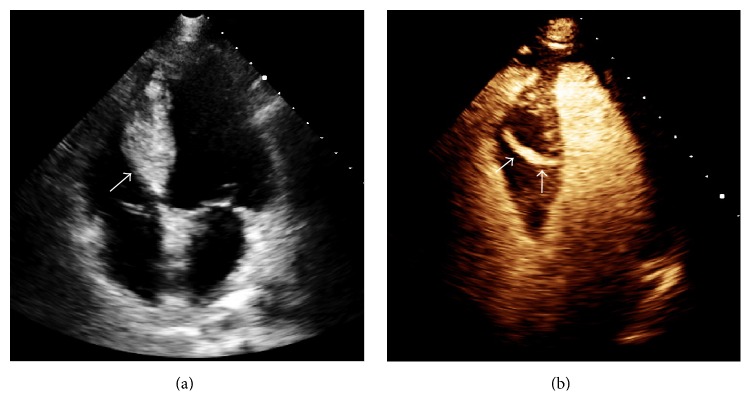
Interventricular septal hematoma (5.3 × 2.6 cm) (arrow, (a)), with coronary-ventricular fistula (5 mm in diameter) (arrows, (b)). (Videos 1 and 2 show baseline echocardiographic findings and are included in Supplementary Material available online at http://dx.doi.org/10.1155/2016/8750603.)
